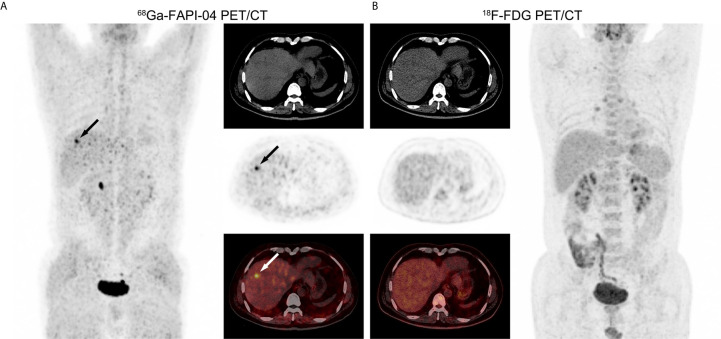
PET/CT images in a 53-year-old male patient with moderately-differentiated HCC. (A)
